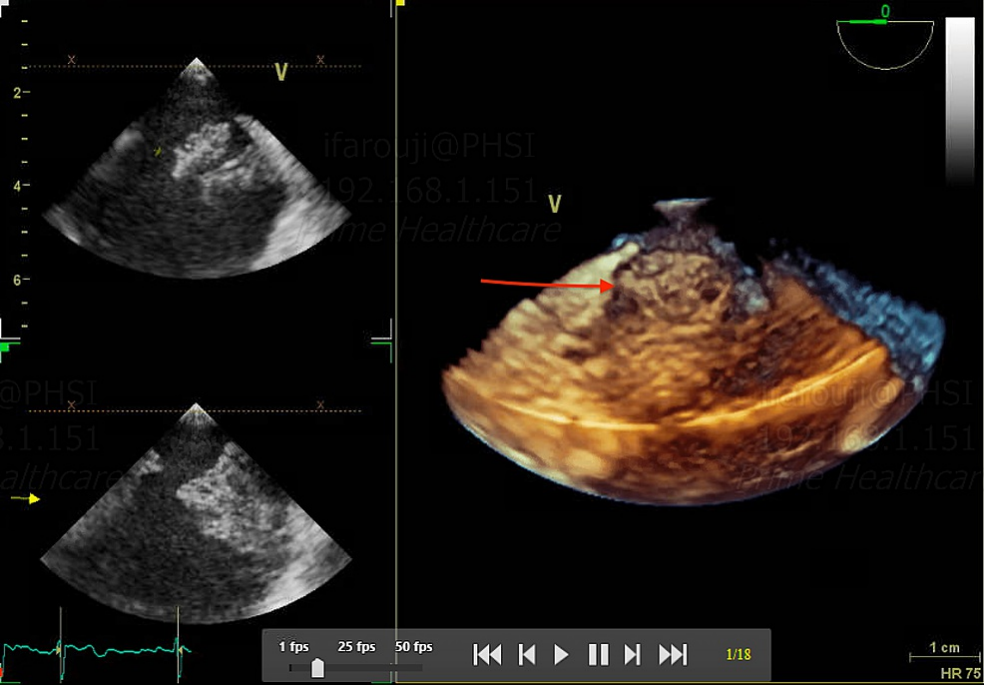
TEE, 3D reconstruction, showing a thrombus attached to the interatrial septum TEE: transesophageal echocardiogram
